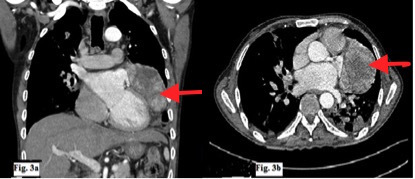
(a,b) Coronal reformatted and axial contrast-enhanced CT image showing left-sided pericardial lesion with heterogeneous enhancement. Non-enhancing hypodense areas are seen within the lesion suggestive of necrotic areas. No obvious invasion of underlying cardiac structures is seen.
